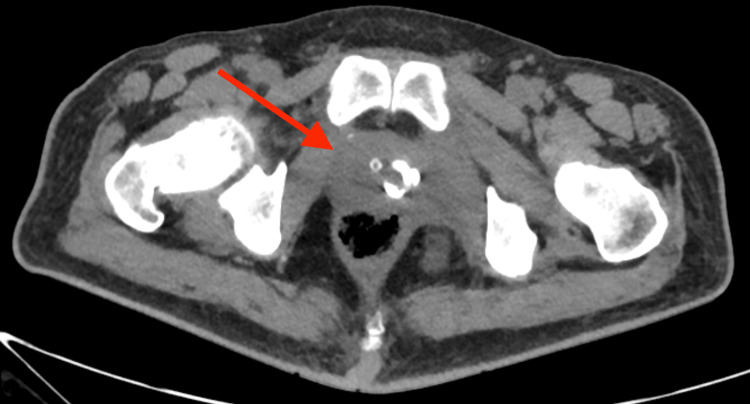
Follow-up non-contrast computed tomography; complete resolution of abscess; transrectal drainage catheter was removed soon afterward
